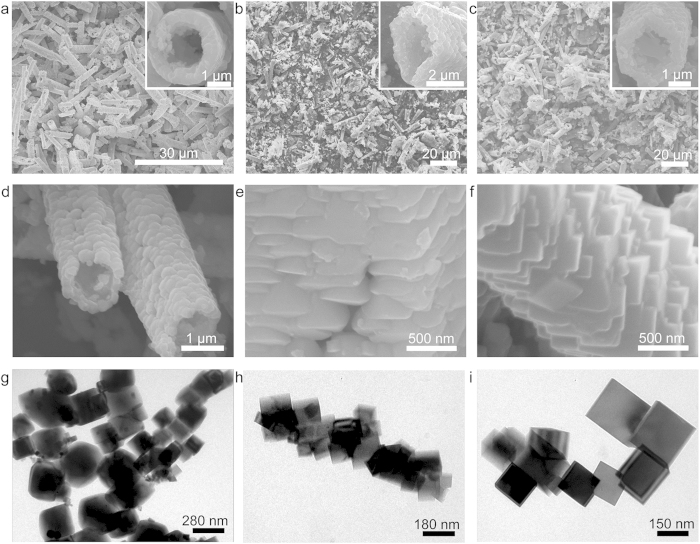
The temperature-dependent morphological evolution of as-synthesized Ni_x_Co_3-x_O_4_ products. Low-magnification SEM images of (a) circular microtubes, (b) square microtubes, and (c) QNHMs prepared by hydrothermal treatment of 0.8 g Ni(NO_3_)_2_∙6H_2_O and 3.2 g Co(NO_3_)_2_∙6H_2_O in 60 mL of ammonia solution (8.3% w/w) for 24 h at 180 °C, 200 °C, and 230 °C, respectively. The insets in (a), (b) and (c) show the tips of three types of microtubes. SEM images of the walls of (d) circular microtubes, (e) square microtubes, and (f) QNHMs. TEM image of the microtube wall structures which derive from (g) circular microtubes, (h) square microtubes, and (i) QNHMs by exfoliating them in intense ultrasonication treatments for 3 h.
